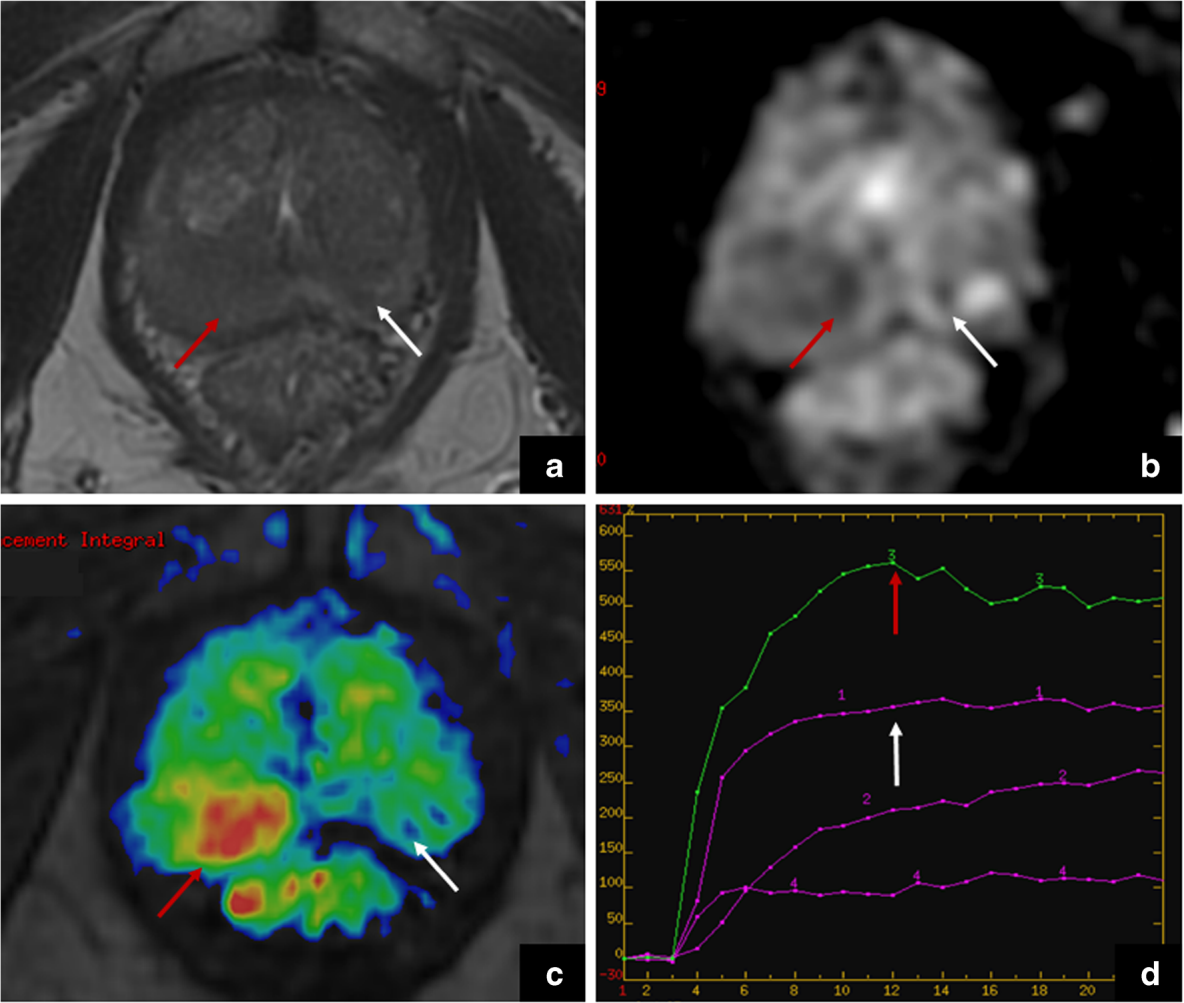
The red arrows show a focal area in the right peripheral zone with low-signal intensity on axial T2-weighted imaging (a), restricted diffusion in the ADC map (b), avid enhancement in the DCE study (c) and an early wash-in curve (d); these findings are consistent with prostate cancer (Gleason 3 + 3). The white arrows show a diffuse area of decreased signal in the left peripheral zone on T2-weighted imaging (a), and a mild restriction in the ADC map (b) and diffuse contrast uptake (c and d); these findings are consistent with prostatitis
